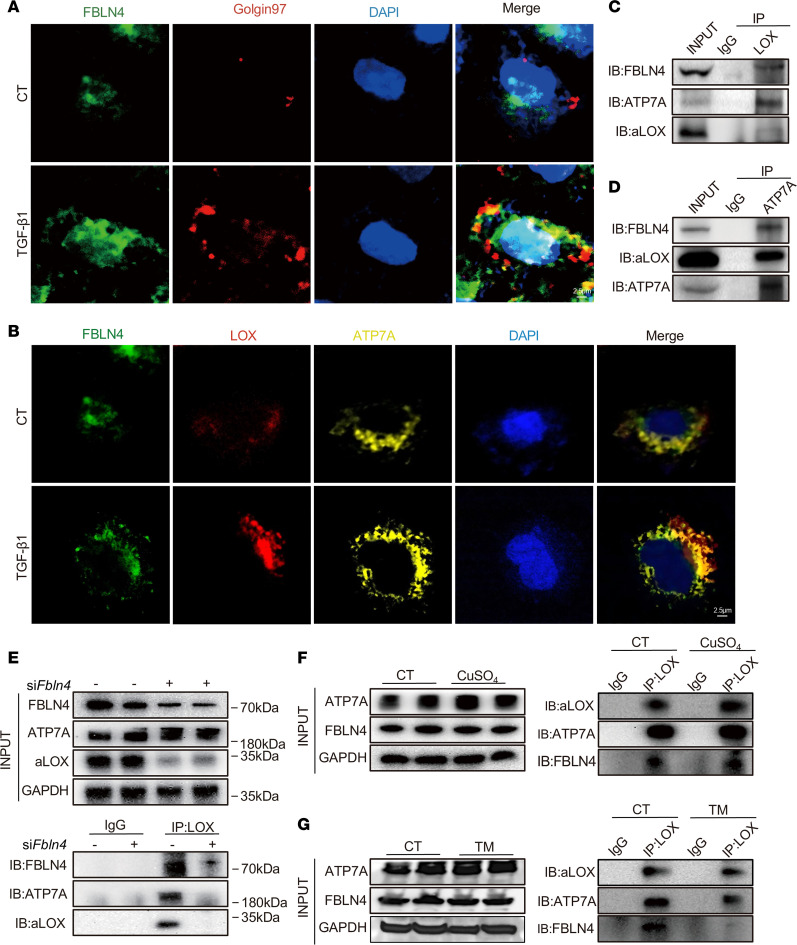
FBLN4 is essential for the formation of the ATP7A-LOX complex in renal fibrosis. (A) The colocalization of FBLN4 (green) with the Golgi apparatus (red) was analyzed by immunofluorescent costaining in NRK-52E cells without (CT) or with TGF-β1 treatment (*n* = 3). Original magnification, ×1,000. Scale bar: 2.5 μm. (B) The colocalization of FBLN4 (green) with ATP7A (yellow) and LOX (red) was analyzed by immunofluorescent costaining in NRK-52E cells without (CT) or with TGF-β1 treatment (*n* = 3). Original magnification, ×1,000. Scale bar: 2.5 μm. (C and D) Coimmunoprecipitation showing the interaction among ATP7A, FBLN4, and aLOX proteins in NRK-52 cells. (E) Coimmunoprecipitation showing the interaction among ATP7A, FBLN4, and aLOX after downregulating *Fbln4*. (F and G) Coimmunoprecipitation showing the interaction among ATP7A, FBLN4, and aLOX proteins in NRK-52 cells under CuSO_4_- and TM-treated conditions. INPUT indicates total protein. IP indicates the antibody used to pull down the interacting proteins. IgG represents the negative control.
